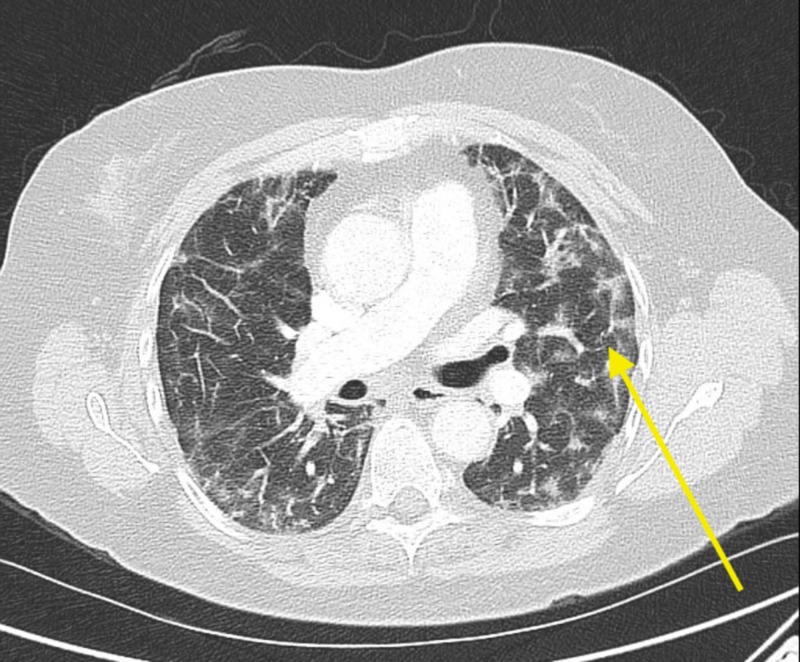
A computed tomography (CT) scan showing opacities described as pneumonia along with patchy infiltrates (arrow).
